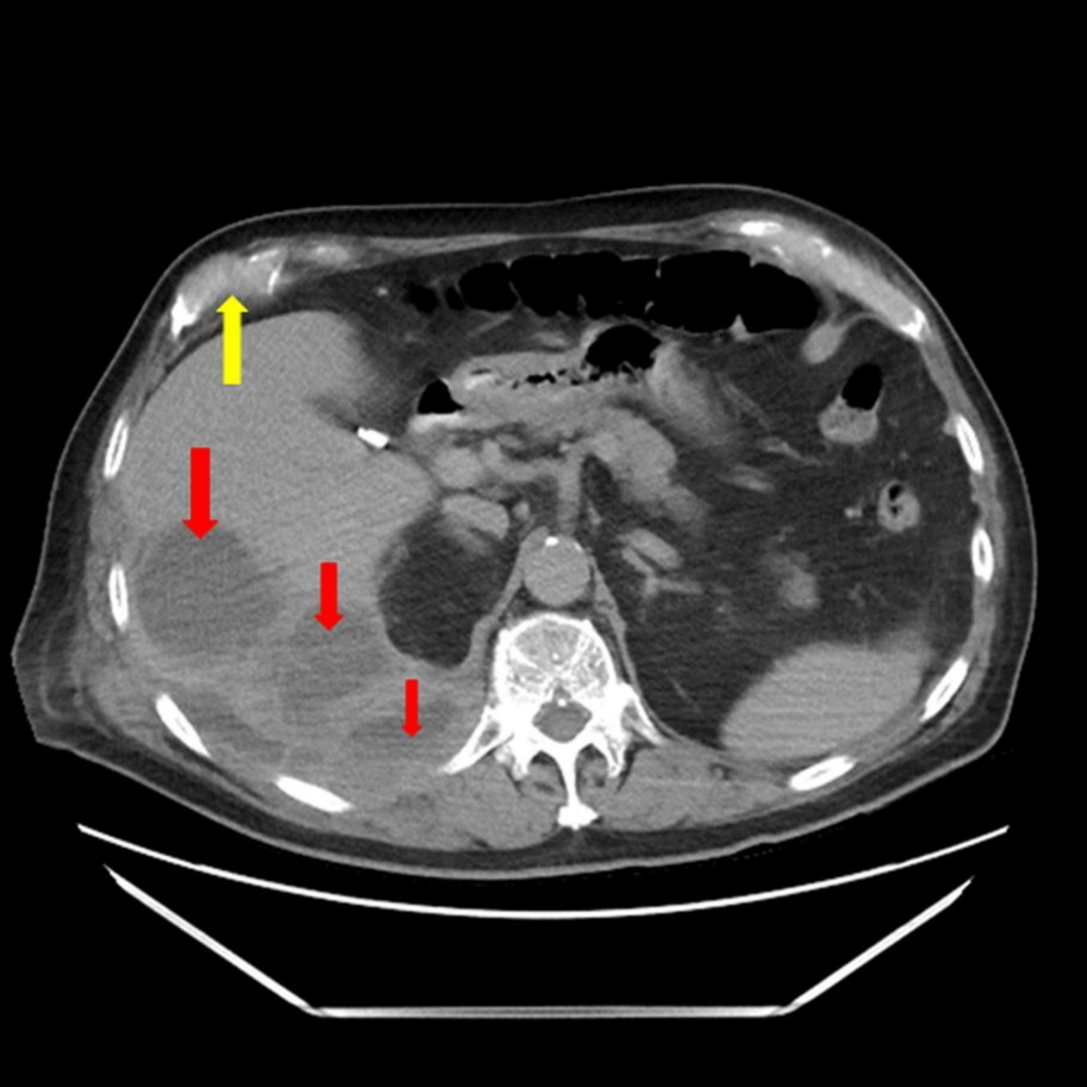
Abdominal computed tomography scan shows a diminished right retro-peritoneal collection inferior and posterior to the right hepatic lobe (red arrows), with a right-sided partially loculated pleural effusion (yellow arrow)
